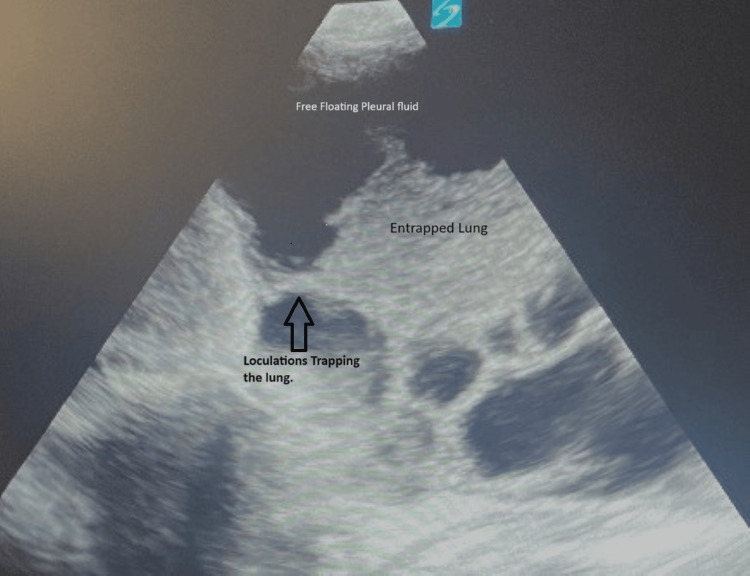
Right-sided lung US showing loculated effusions and multiple septations US: ultrasound
